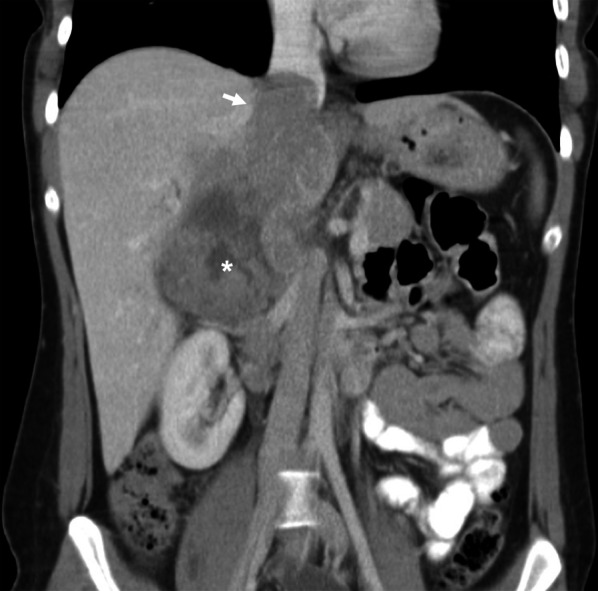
Adrenal cortical carcinoma invading IVC. Portal venous CT coronal image demonstrates a large adrenal cortical carcinoma (asterisk) with irregular margins, areas of necrosis and invasion of the IVC (arrow)
